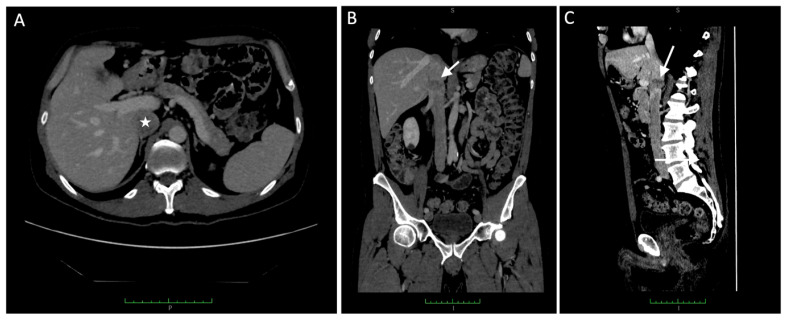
Contrast-enhanced computed tomography of the upper abdomen reveals a heterogeneously enhancing soft tissue mass originating from and expanding the lumen of the inferior vena cava (IVC), as observed on (A) axial section (with asterisk), (B) coronal section (with white arrow), and (C) sagittal section (with white arrow).
